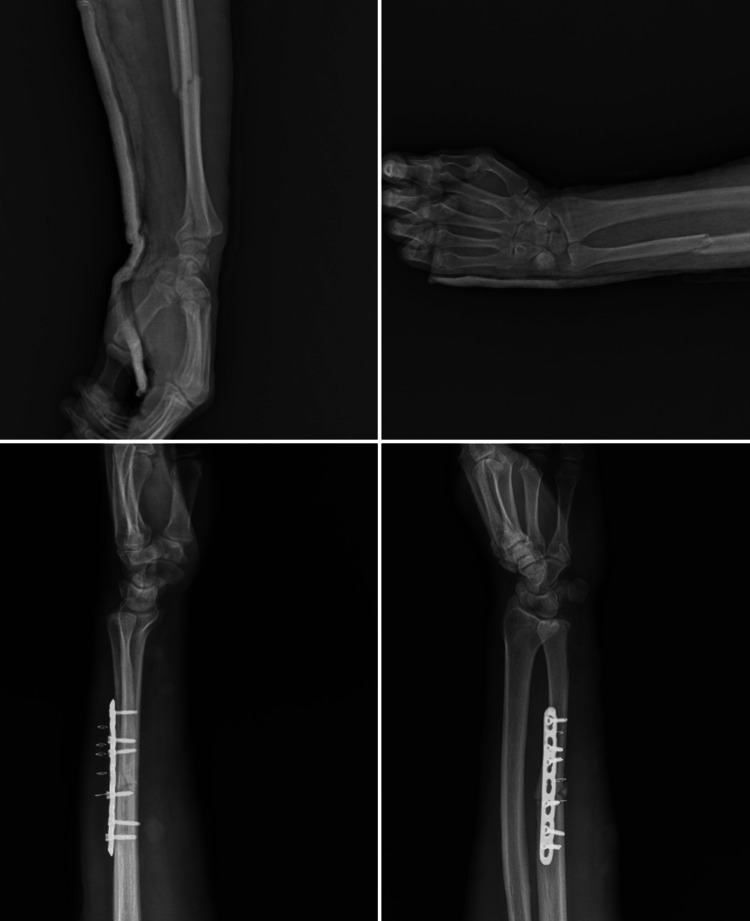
Preoperative and postoperative X-ray images of the patient.
